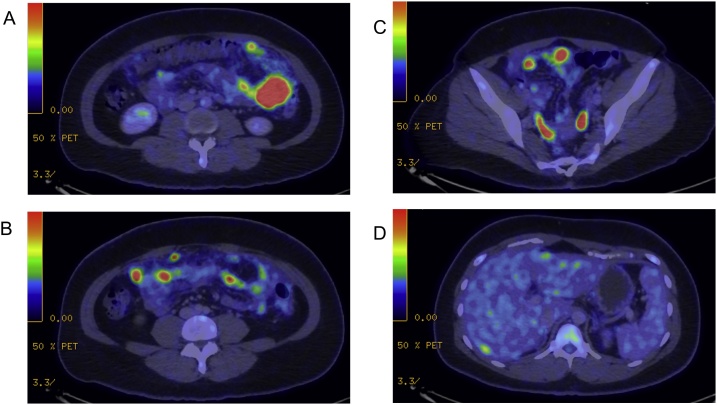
PET-CT scan images showing disseminated FDG-avid foci in A) Omentum, B) Small bowel, C) Pelvis and D) Increased peripheral liver uptake.
